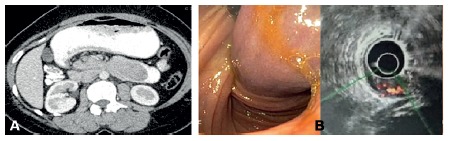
A) CT image shows an intraluminal polypoidal lesion in second part of duodenum with mild duodenal wall thickening; B) endoscopy with EUS shows a large mixed echogenic submucosal lesion of size 4.0x2.8 cm with a 2 cm thick, long pedicle and a feeding artery on color Doppler leading to duodenal intussusception
